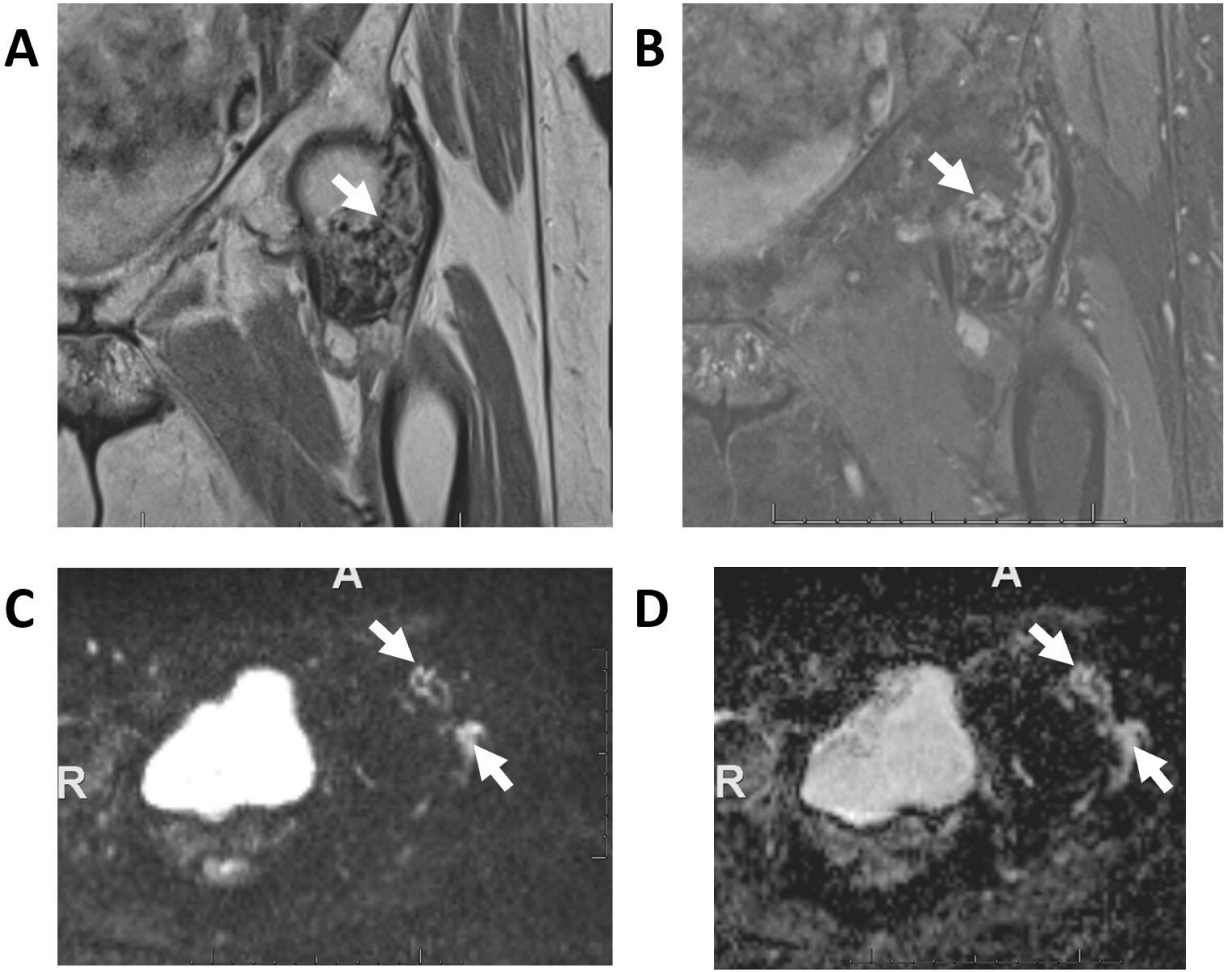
Synovial osteochondromatosis (arrows). (A, B). Coronal T2 Dixon (in-phase) and water maps show multiple similar-sized bodies consistent with SOC. Corresponding DWI (C) and ADC (D) images show T2 shine through with bright SI on both images. ADC, apparent diffusion coefficient; DWI, diffusion-weighted imaging; SI, signal intensity; SOC, synovial osteochondromatosis.
